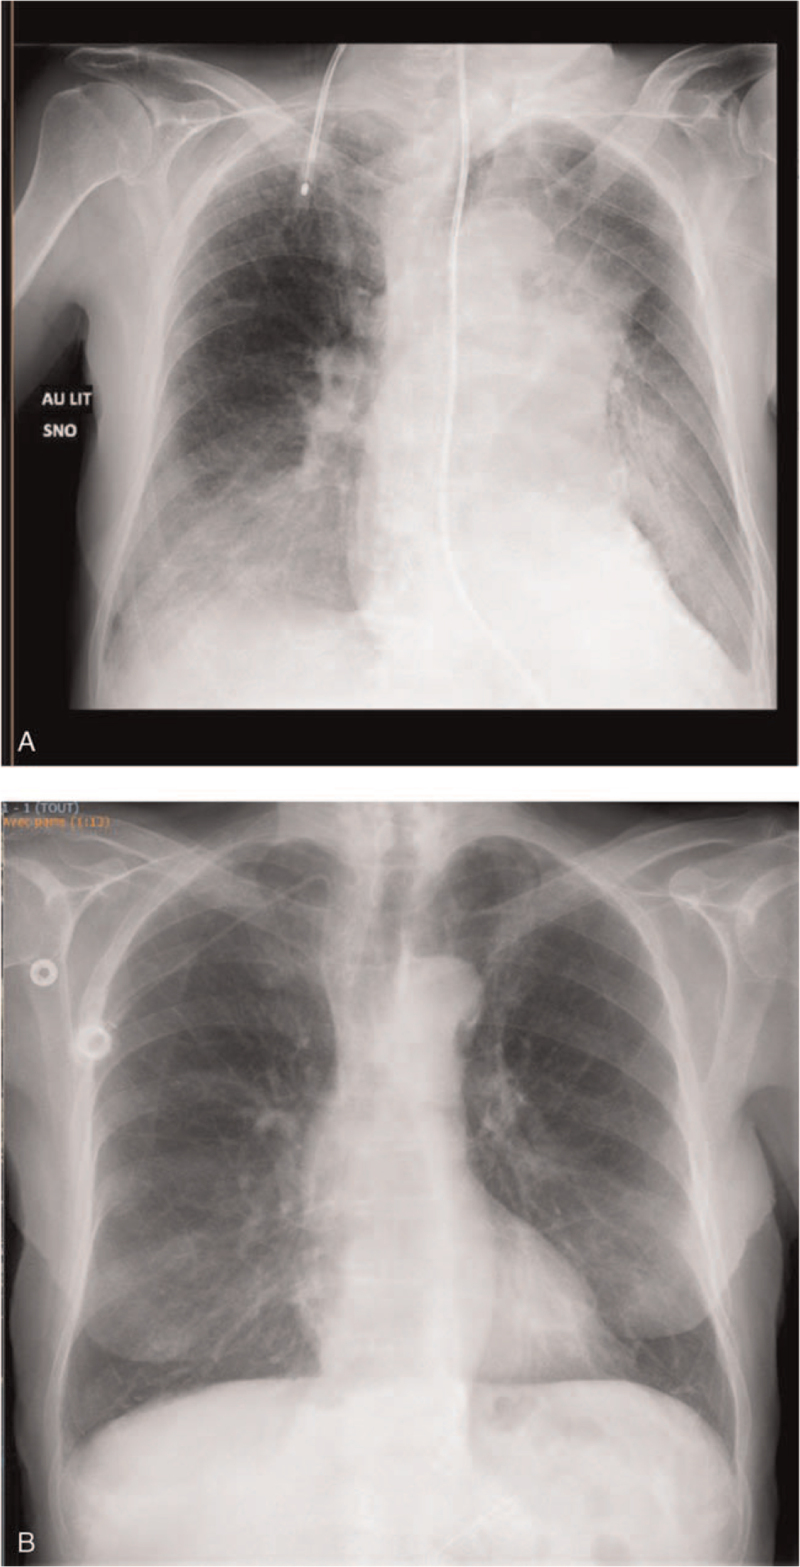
Tumor assessment by chest X-ray at baseline (A) and after 3 months of treatment with alectinib (B).
